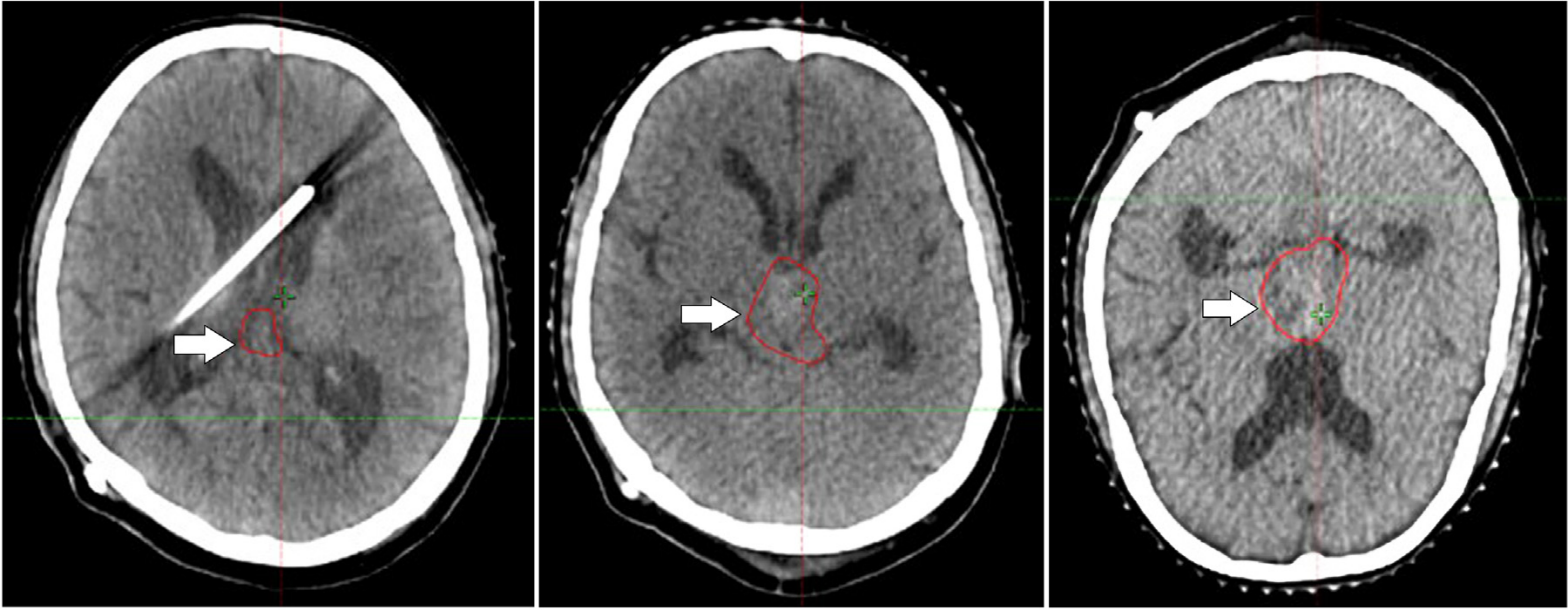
Postoperative radiotherapy planning CT Axial images showing the residual tumor in the pineal region after the subtotal resection of the tumor.
